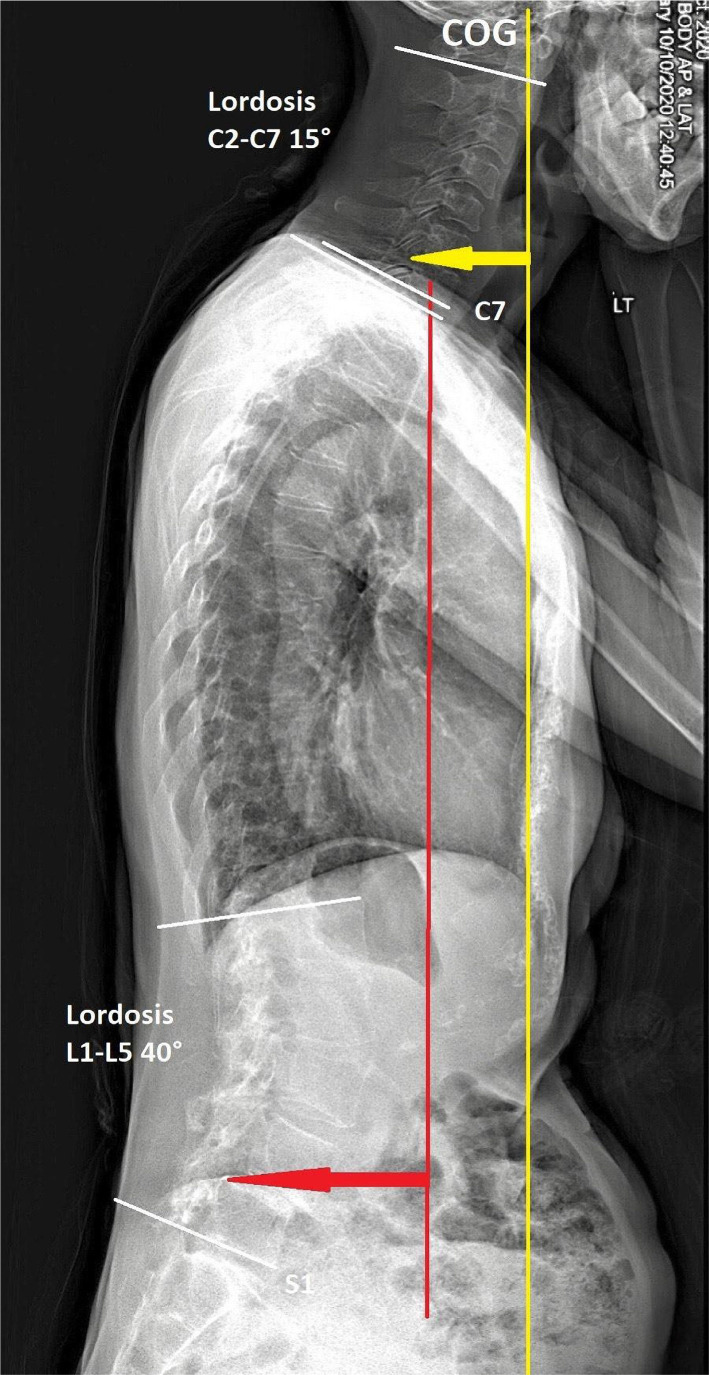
Postural parameters assessed on a sagittal standing full-spine EOS® x-rays: Initial radiograph identified a forward head, stooped posture, and reduced cervical lordosis. The center of gravity (COG) of the head was shifted anteriorly.
